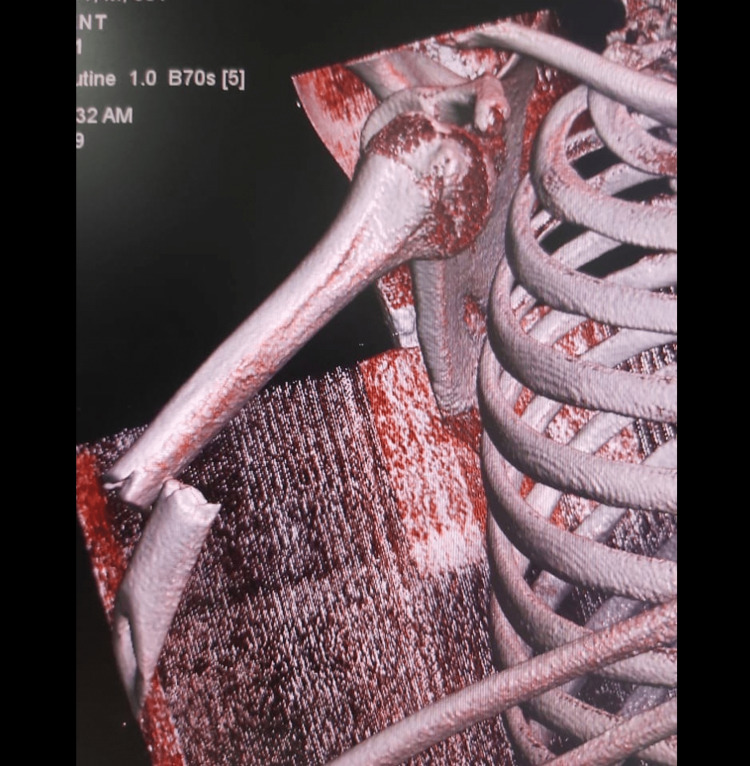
CT scan 3D showing anterior dislocation of the right shoulder with a mid-shaft fracture of the humerus
